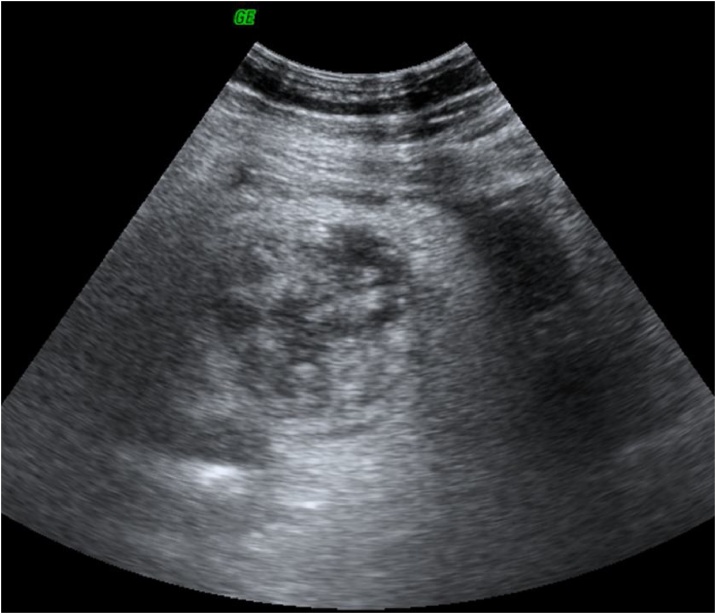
Ultrasound picture (using a low-frequency probe) showing a low echogenic, heterogeneous, thick-walled regular formation with a significant infiltration of the adjacent area.
